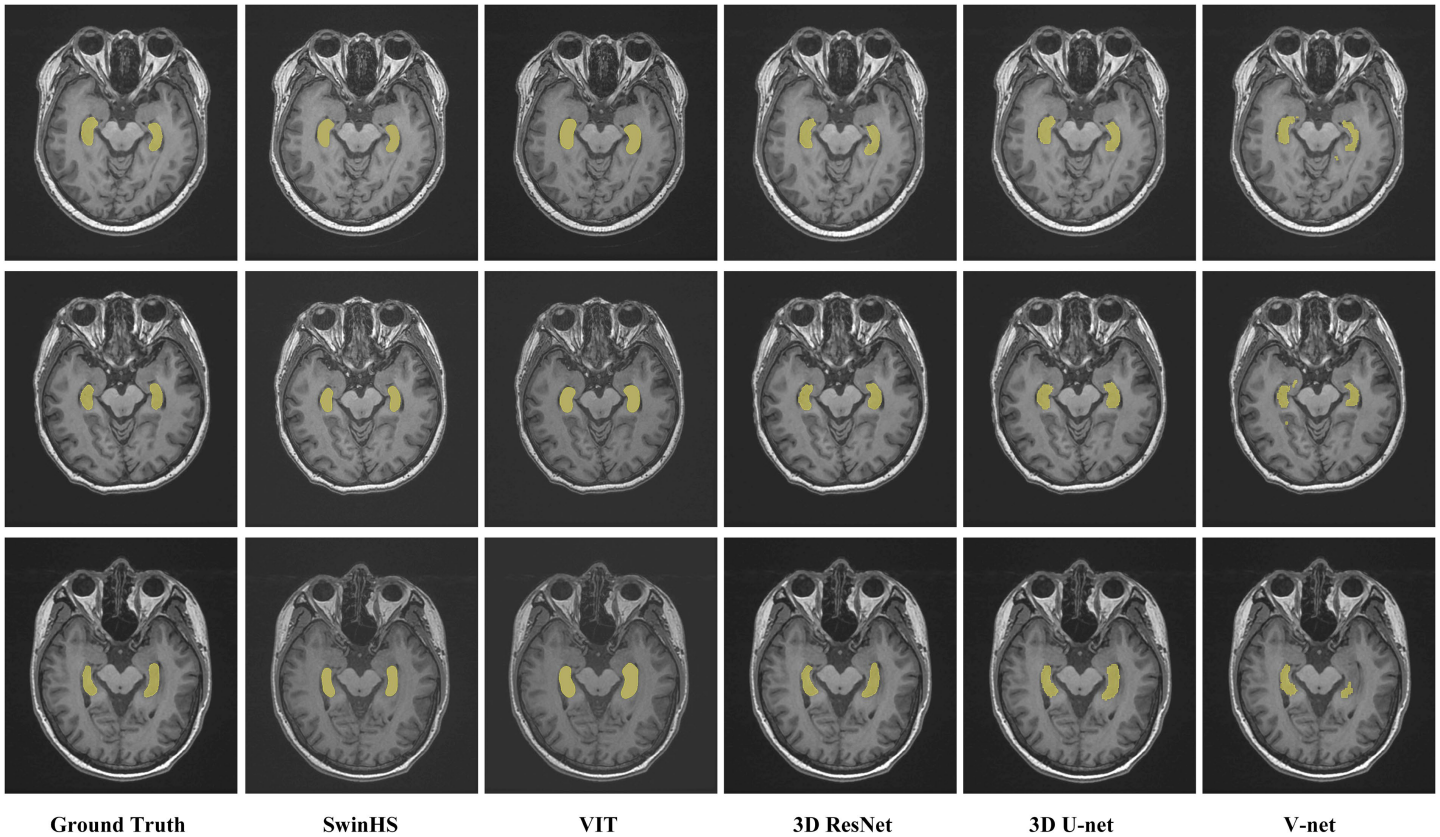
Representative cases of the proposed model and other model methods. The first column shows the contour of the hippocampus in the real MR image. Columns 2 to 6 show the results of the proposed model, VIT, 3D ResNet, 3D U-Net, and V-Net, respectively.
